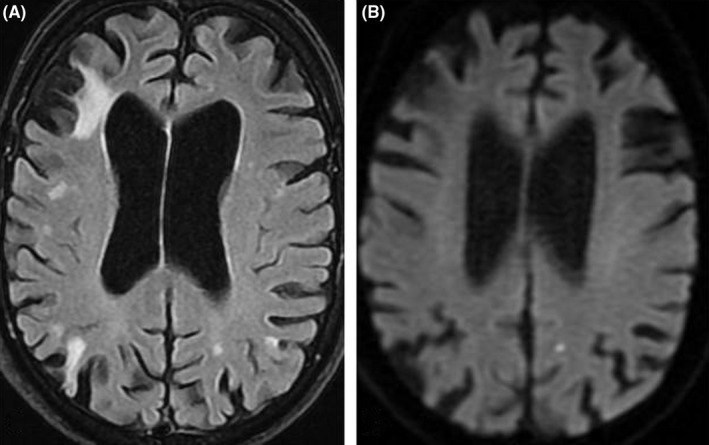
MRI on current admission. A, Multiple cortical and subcortical infarct sequelae on MRI FLAIR. B, Acute microinfarct on diffusion‐weighted MRI (DWI)
